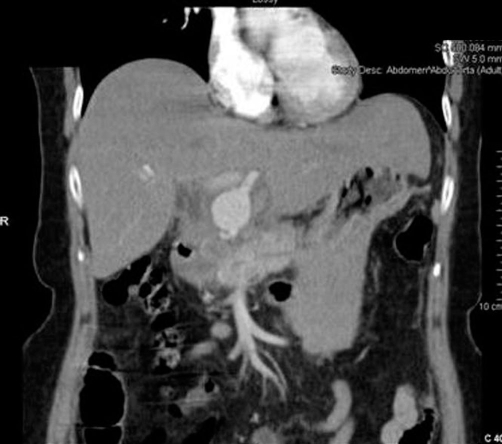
Computed tomography demonstrating left hepatic artery aneurysm.
